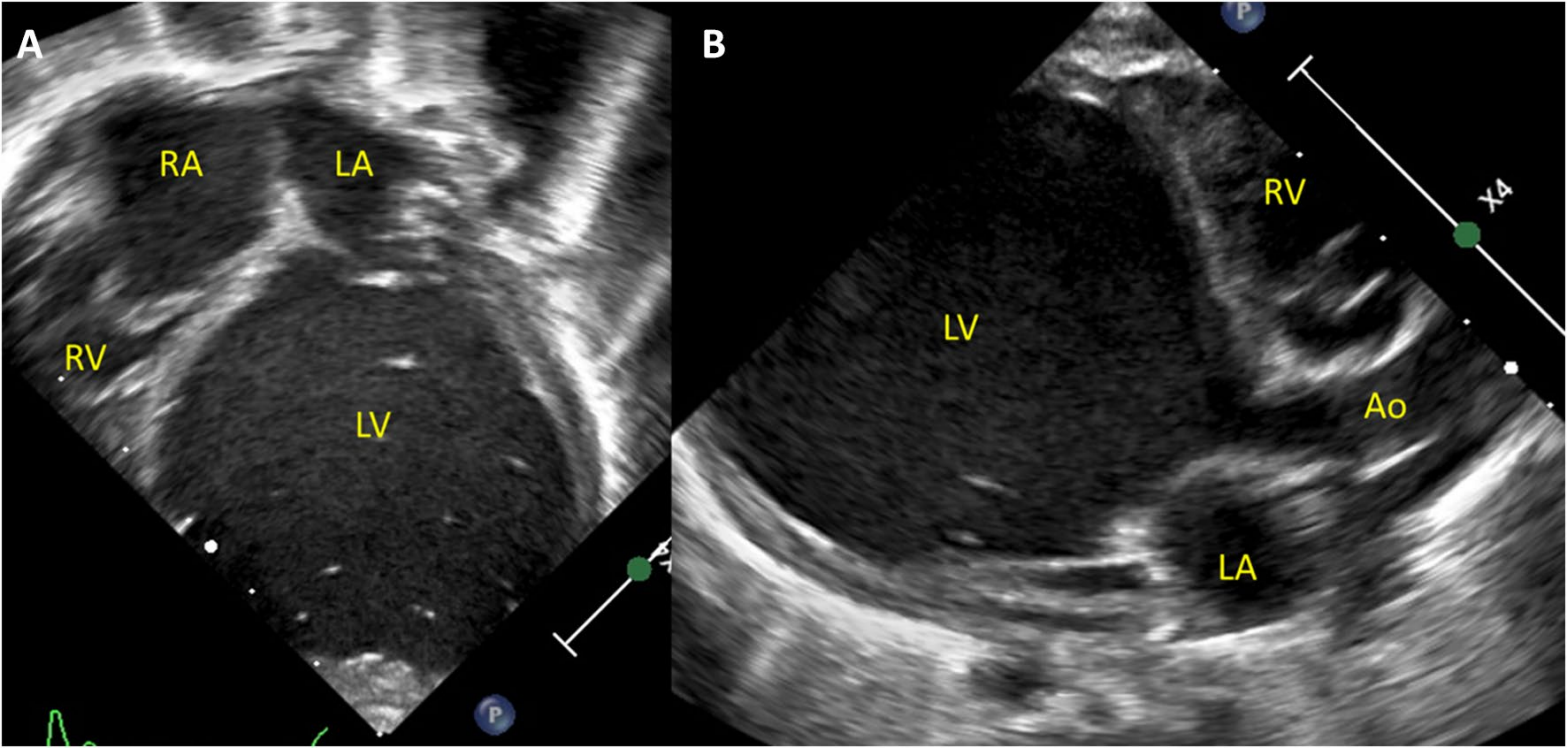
Apical 4 chamber (A) and parasternal long axis (B) echocardiogram images of patient 6 showing a severely remodeled left ventricle with severe dilation and myocardial thinning. The right ventricle is normal in size
